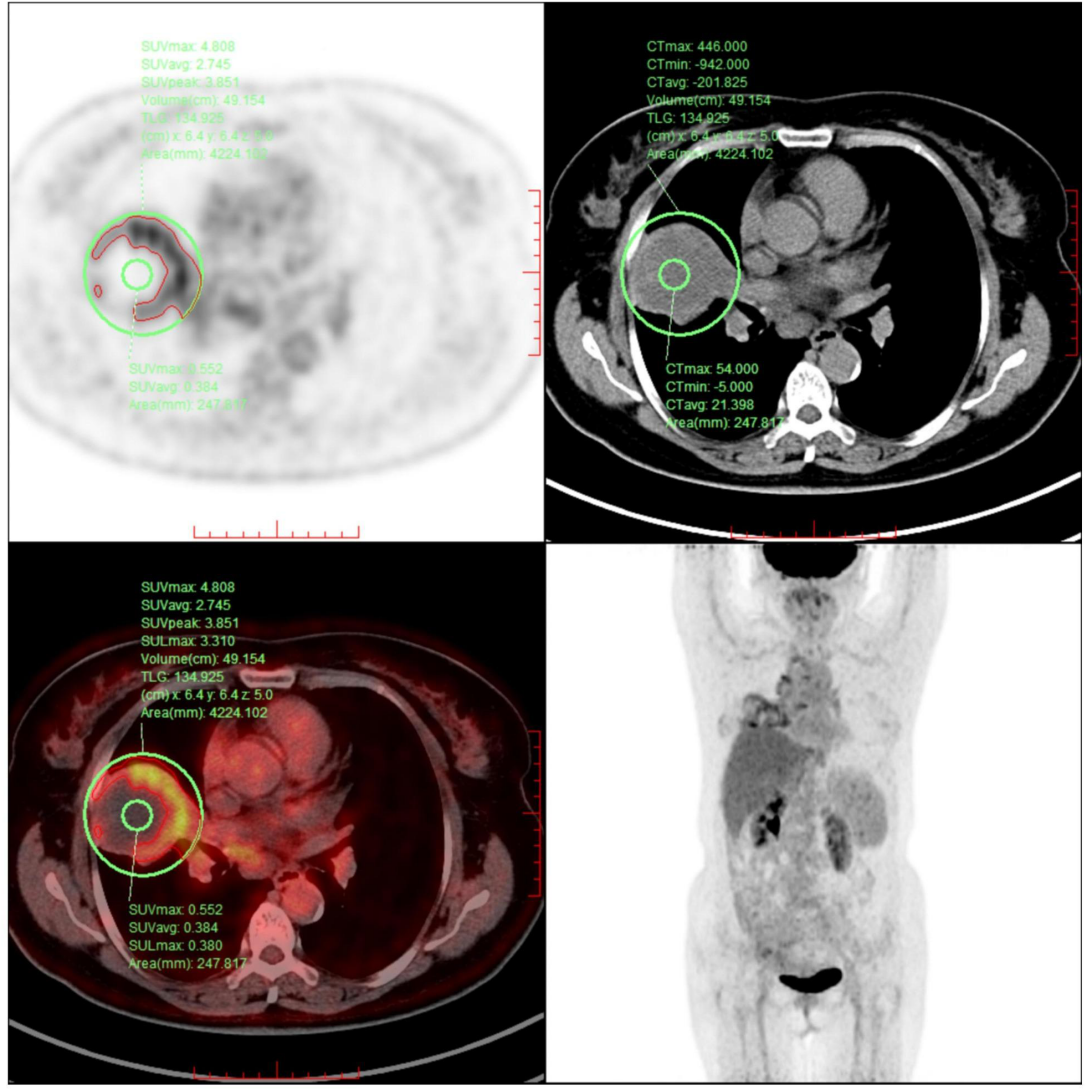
The image reveals a ring-shaped pattern of increased FDG uptake, with higher standardized uptake values (SUV) observed at the periphery of the lesion compared to its center.
